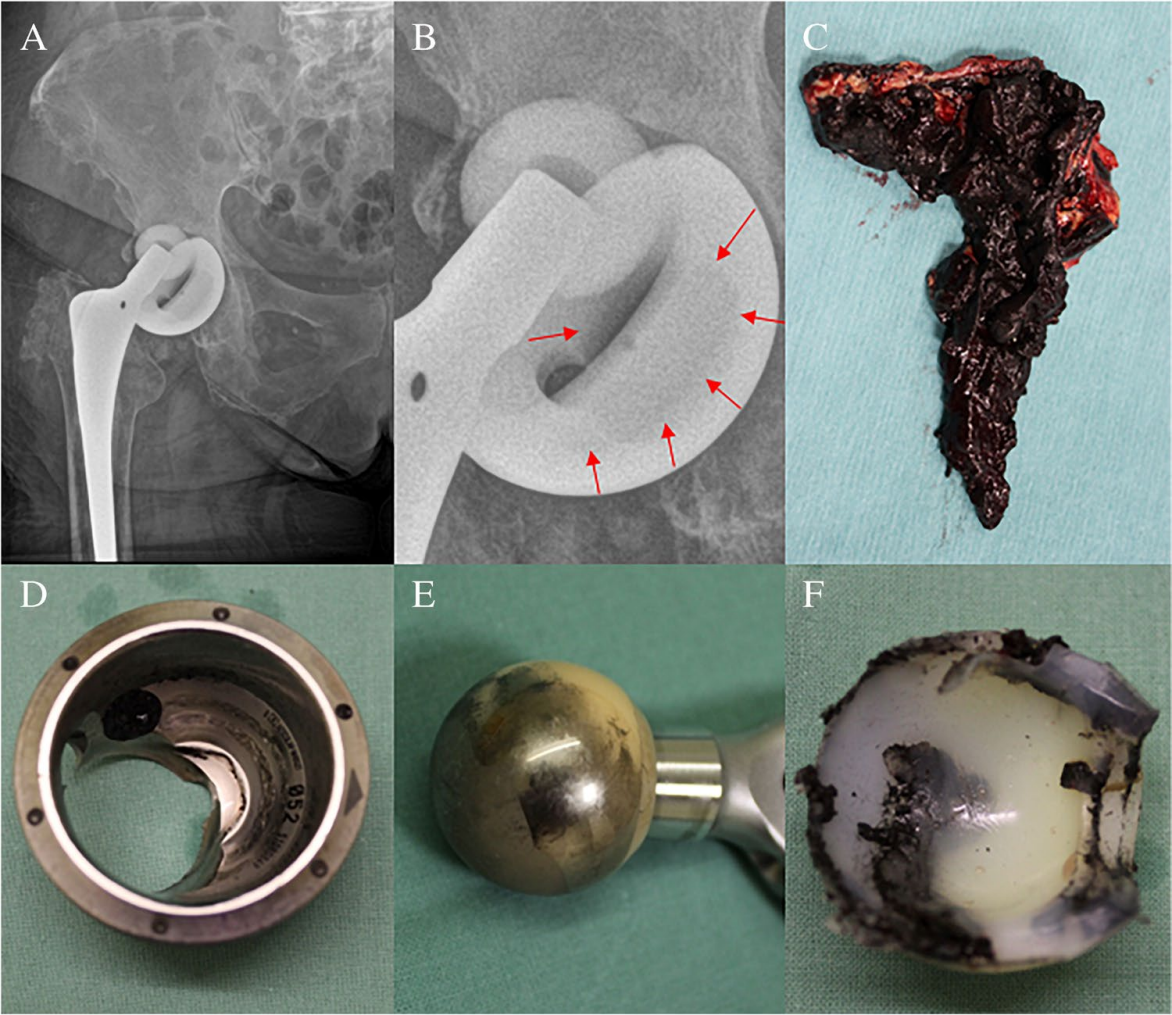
X-ray of the right hip of an 81-year-old female with a loosened cup (A) which is at some part wear-through (B); sample of periprosthetic tissues (C), the intraoperative picture of the removed metallic cup (D), ceramic head (E), and damaged polyethylene liner front side (F)
